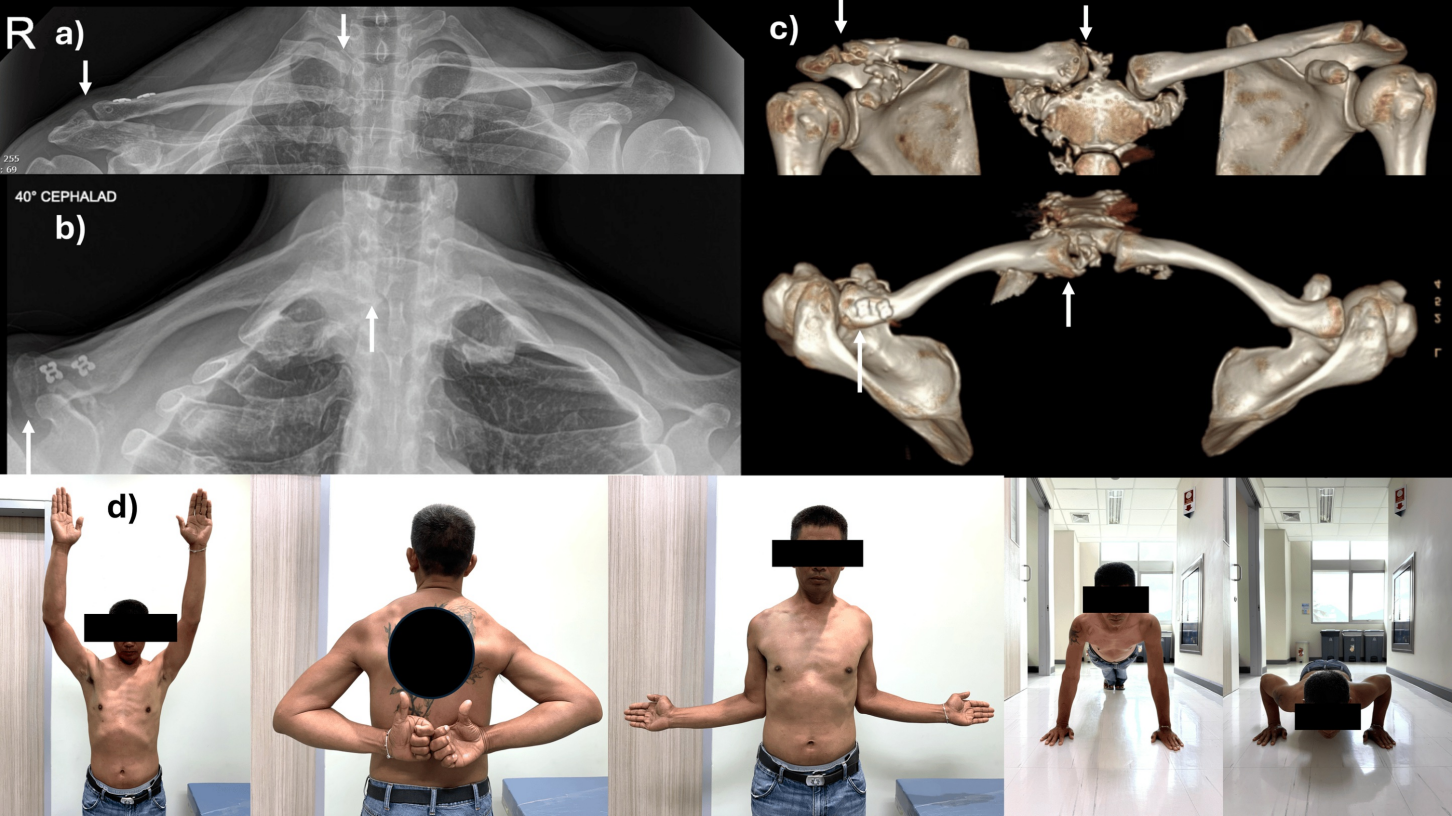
Postoperative radiographic and clinical examination of the patient. (a and b) Radiographs of both clavicles in the anteroposterior and cephalad tilt views. (c) 3D CT scan of both clavicles. (d) The clinical function of the patient at one-year follow-up. The arrow showed reduced acromioclavicular and sternoclavicular joints.
